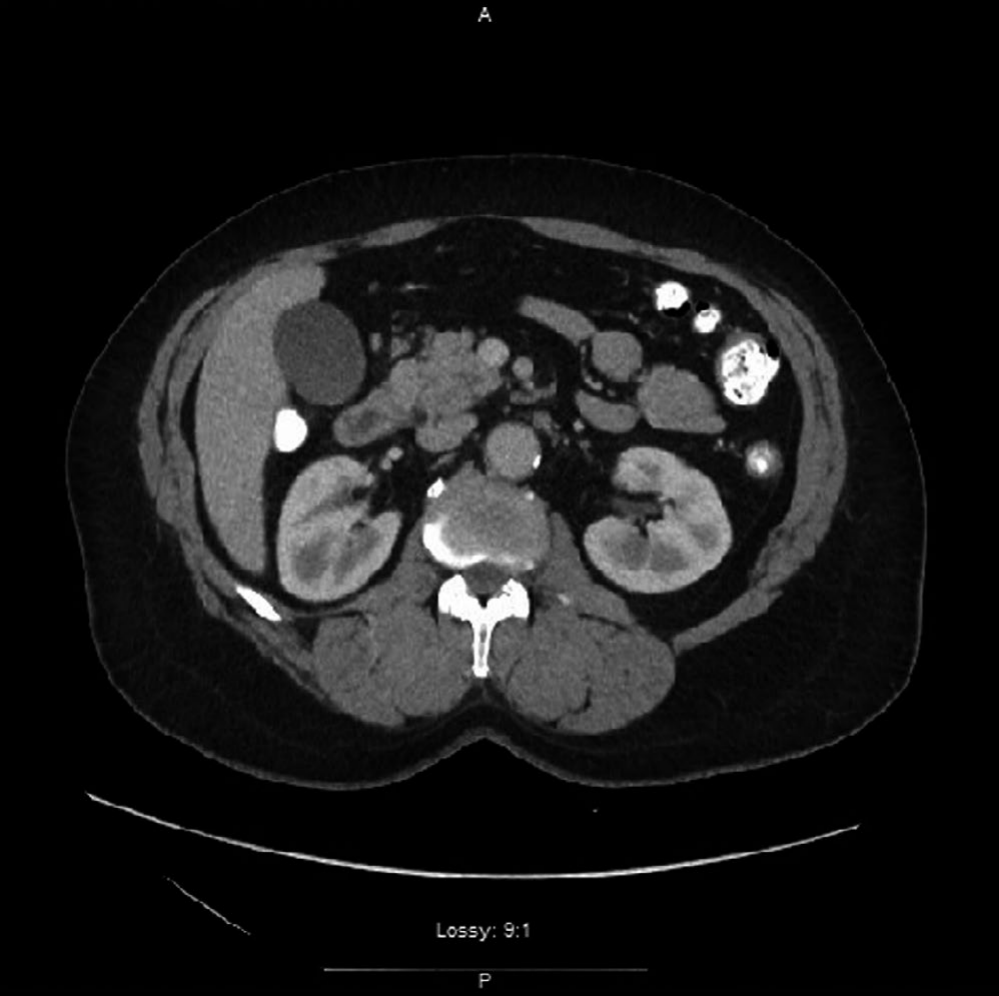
CT Abdomen & Pelvis with contrast demonstrating inflammation surrounding the middle portion of the duodenum and head of the pancreas suggesting acute pancreatitis. (Axial View)
